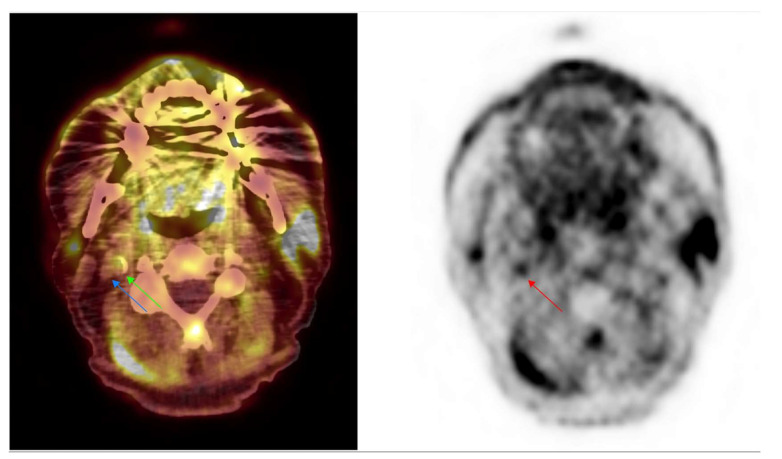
Incidental findings from atherosclerosis in [^18^F]FMCH PET/CT imaging. Axial [^18^F]FMCH PET/CT performed in a 66 year old patient to assess prostate adenocarcinoma disease. Images showed an intense focal uptake (red arrow) on PET imaging with an hyperdensity on PET/CT (green arrow) corresponding to an atherosclerosis plaque with mineralization from the right internal carotid. The internal vein is shown (blue arrow).
